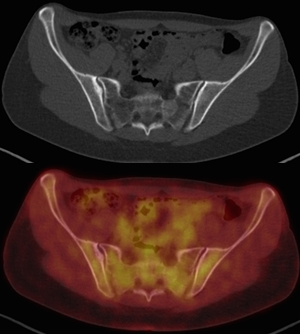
PET-CT scan showing a well-defined sclerotic lesion with mild fludeoxyglucose avidity (average standardized value 2.5) in the S2 segment. No other abnormalities were found on PET-CT scan. PET, positron emission tomography.
